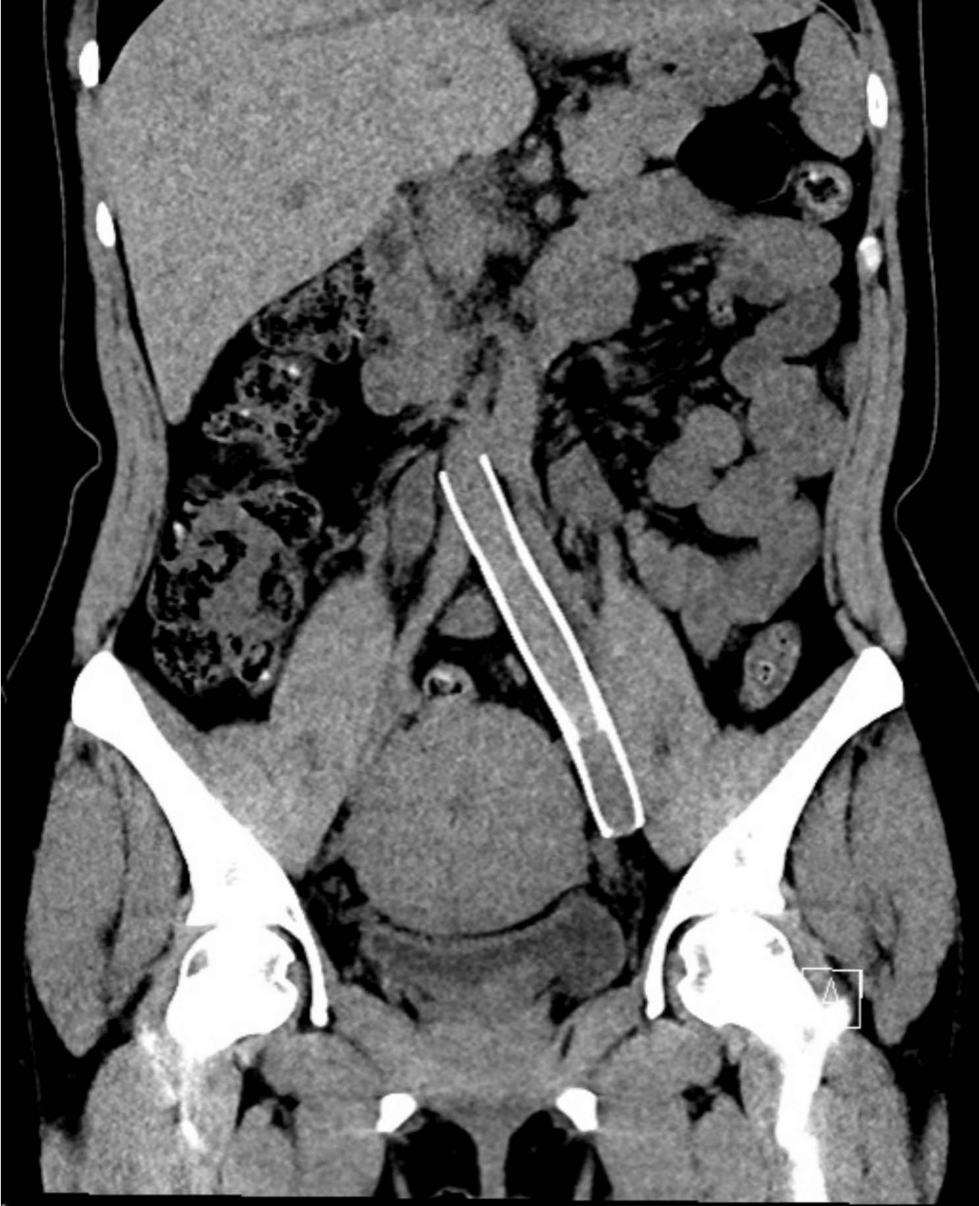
Coronal CT view of the stented LCIV. CT: computed tomography; LCIV: left common iliac vein
